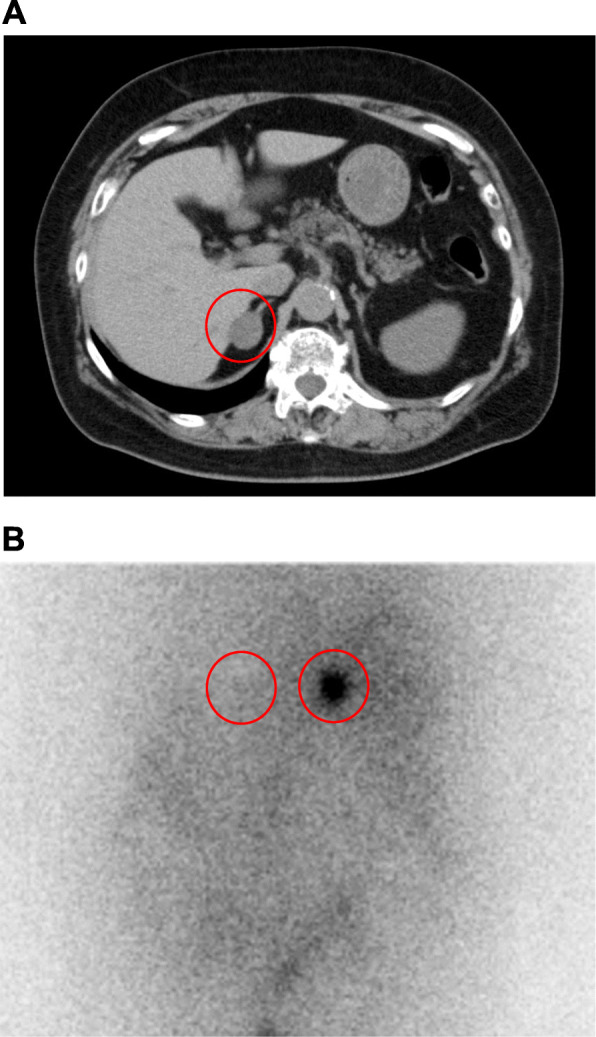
A In abdominal computed tomography, tumorous lesion was observed in right adrenal gland. Its size was as large as 25 mm × 22 mm. B In adosterol scintigraphy, high accumulation was observed in the same area, whereas there was no accumulation at all in the other side
